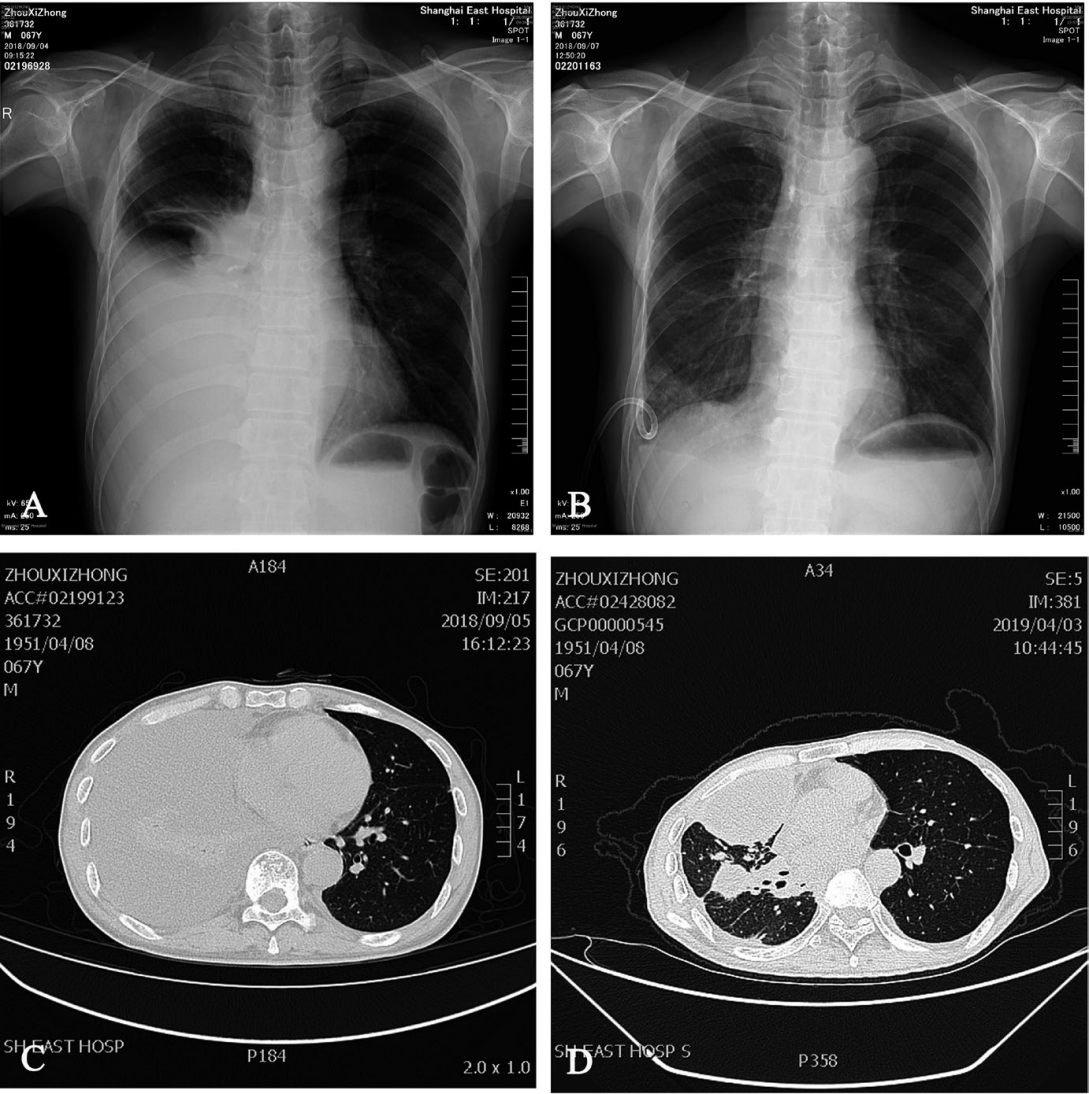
A 67‐year‐old male patient underwent IPHC by VATS and diagnosed with lung adenocarcinoma and MPE. (A) A chest radiograph showed a large pleural effusion before treatment. (B) A chest radiograph showed the pleural effusion was cured after IPHC. (C) A CT imaging showed that a large pleural effusion before treatment. (D) A CT imaging showed that a small amount of encapsulated pleural effusion after 6 months. IPHC, intrapleural perfusion with hyperthermic chemotherapy; VATS, video‐assisted thoracoscopic surgery; MPE, malignant pleural effusion
